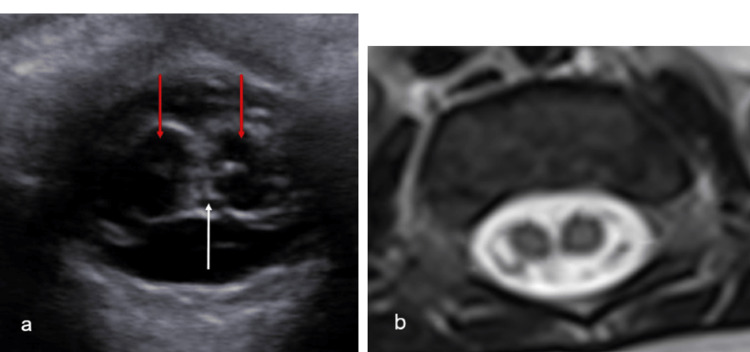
Hemicords (a) Transverse ultrasound image demonstrating two hemicords (red arrows), separated by a thin septum (white arrow). (b) Axial T2-weighted MR image of the same patient confirms the findings Radiological images obtained by the authors
